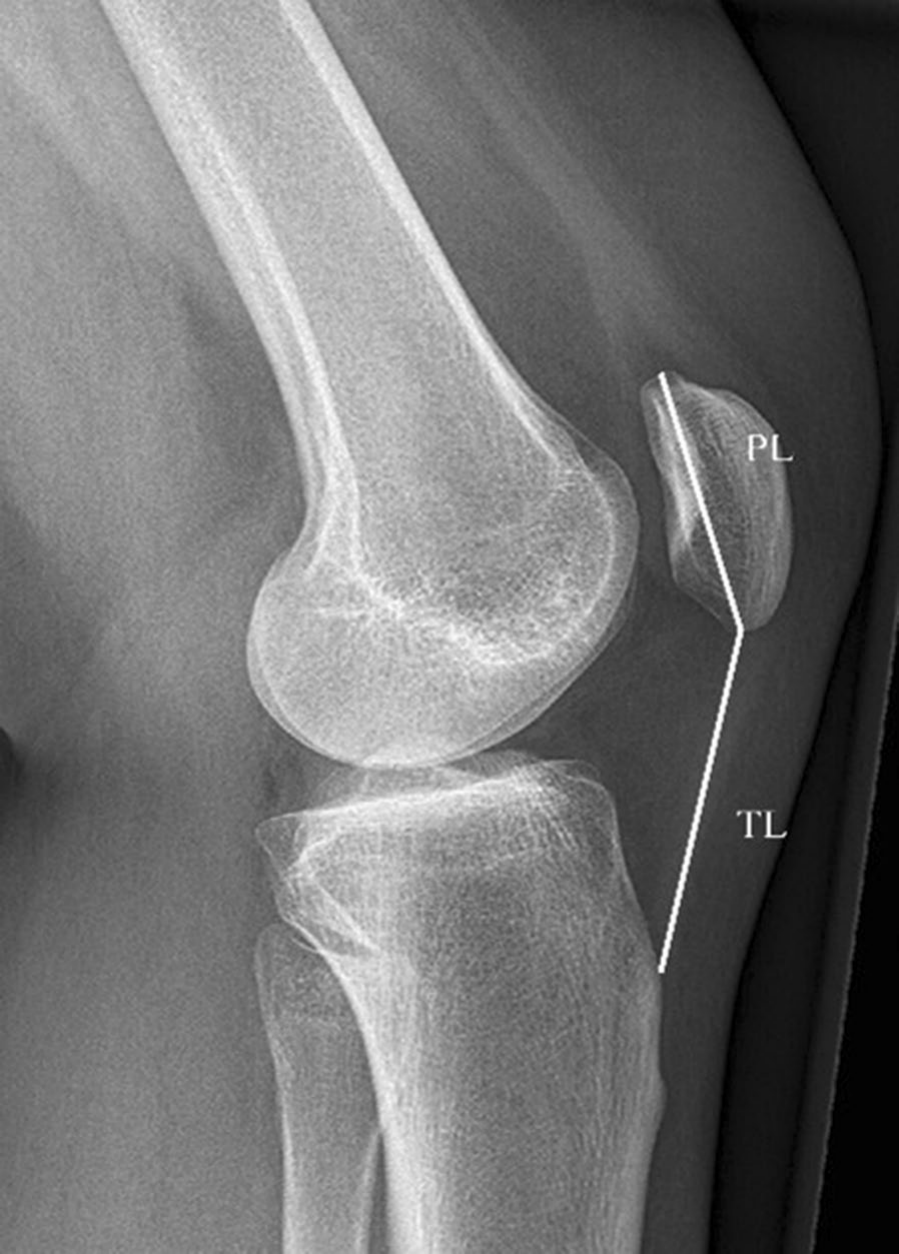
Insall–Salvati ratio. On a 30° flexed lateral knee x-ray, draw two distance lines (*PL* patella length, *TL* patellar tendon length)
